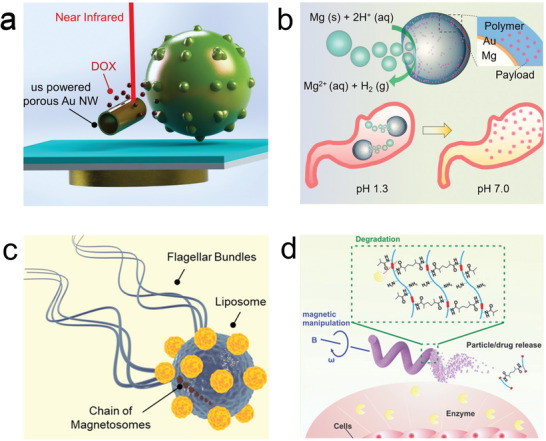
Micro/nanorobot based delivery of pharmaceuticals. a) Ultrasound propelled nanowires for near‐IR triggered delivery of doxorubicin. Reproduced with permission.^[^
